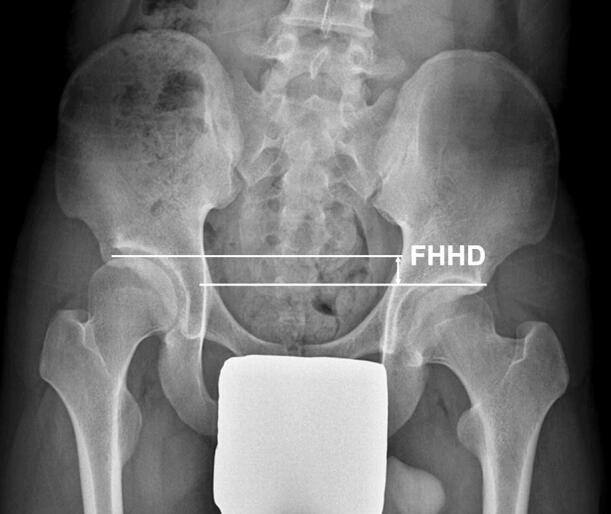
Measurement of femoral head height difference (FHHD) on standing anteroposterior radiograph of the hip.
